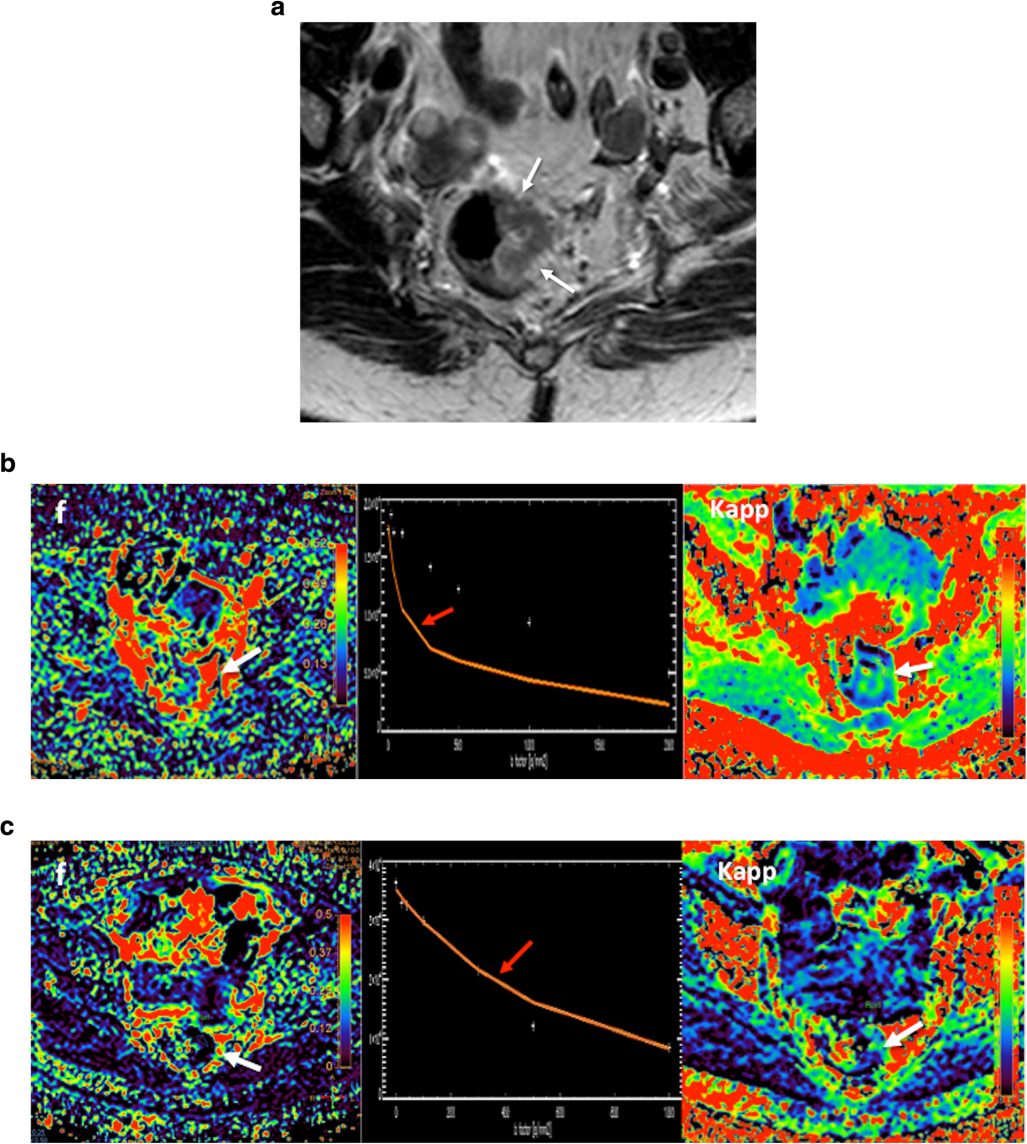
Rectal cancer in a 65-year-old woman. a Axial T2-weighted image demonstrated a rectal mass deeply extending to the mesorectal fat (white arrow). b Perfusion fraction (*f*), apparent kurtosis diffusion (Kapp), and the curve of signal intensity decay in diffusion pretherapy. Note that this curve showed a marked attenuation of signal intensity at low *b* values (red arrow) due to the influence of tumor perfusion on diffusion. c Following therapy, there are reduction of both f and Kapp and dissapearance of the fast attenuation of the signal intensity at low *b* values, related to tumor response with reducer perfusion on IVIM model
